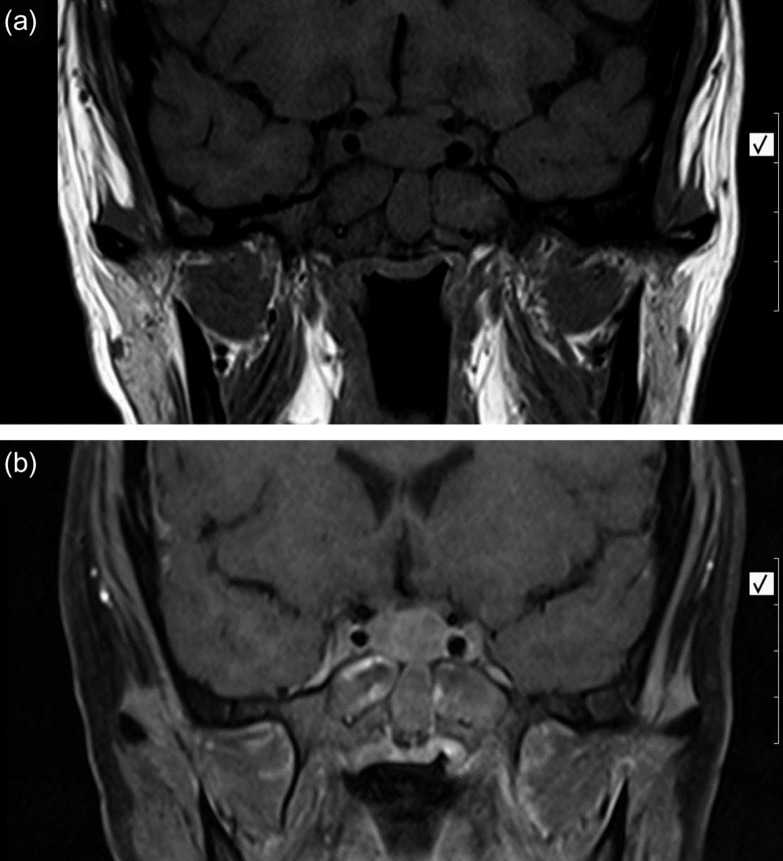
(a) A T1-weighted magnetic resonance imaging (MRI) of the sellar region showing a diffusely enlarged pituitary gland measuring 1.6 (W)×1.1 (H) cm with lateral extensions encasing both cavernous sinuses bilaterally and mild compression of the optic chiasm. (b) A T1-weighted MRI of the sellar region after contrast showing heterogeneous enhancement of the enlarged pituitary gland along with the thickened lateral walls of both cavernous sinuses.
